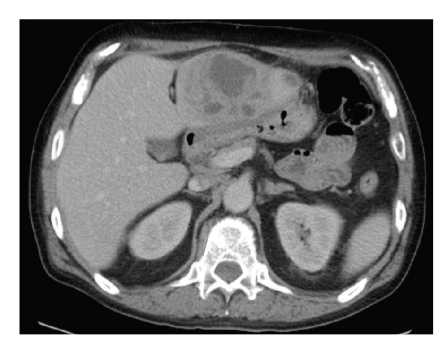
CT demonstrating left liver abscess.
